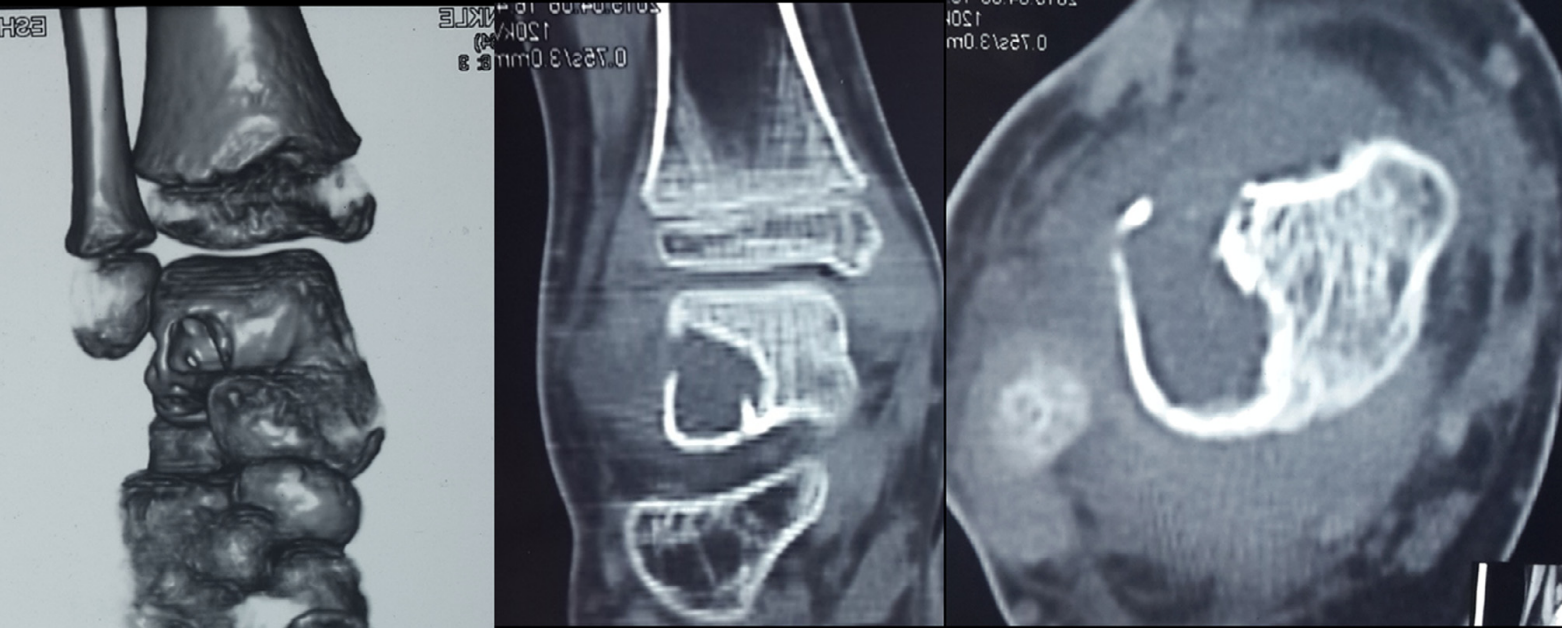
CT scan of an 8 year-old child showing a large aneurysmal bone cyst eroding the anterolateral cortex of talus.
